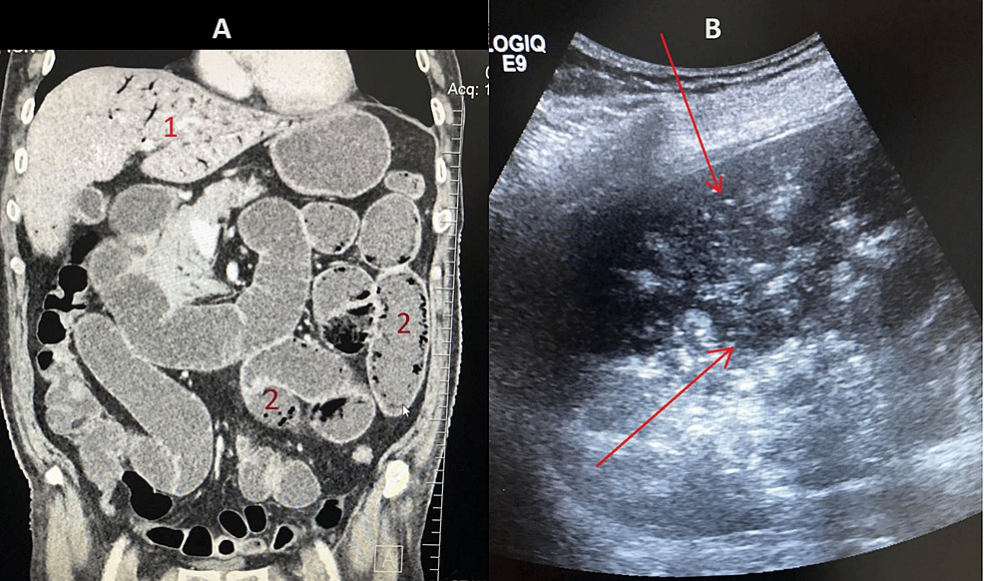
Abdominal/pelvic CT and ultrasonography showing signs of HPVG and small bowel pneumatosis. (A) CT in the frontal plane. 1 - HPVG at the level of the left hepatic lobe. 2 - Jejunoileal pneumatosis. (B) Ultrasound imagery showing multiple portal echogenic foci, indicated by the red arrows. HPVG: hepatic portal venous gas.
